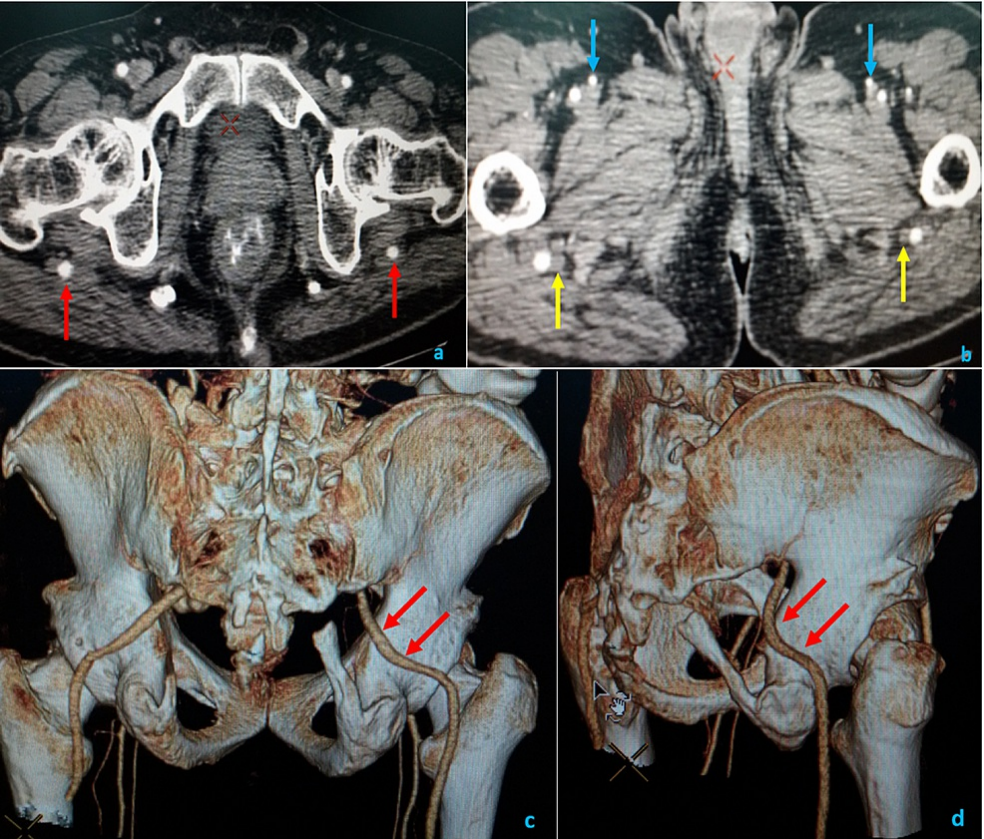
A persistent sciatic artery in a 40-year-old male patient CT angiogram and volume-rendered images showing ischial tuberosity (a), upper thigh (b), posterior projection (c), and oblique projections (d). Also seen are axial limb arteries (red arrows). Arteries are accompanying the sciatic nerves (yellow arrows), bilateral superficial femoral arteries (blue arrows), and persistent sciatic artery (red arrows).
